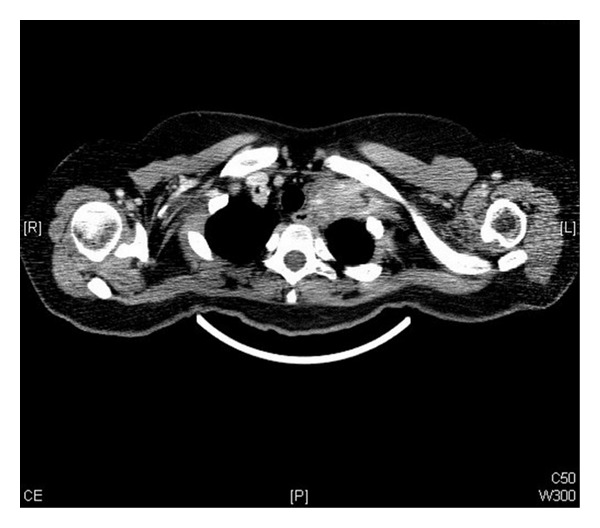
Computed tomography (CT) axial images of neck showing left supraclavicular solid mass of size 3.2 × 2.5 cm encasing the vessels.
